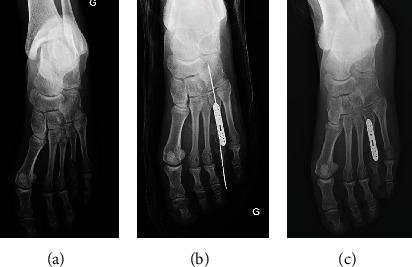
Case 2, a 26-year-old female with bilateral brachymetatarsia of the fourth toes who underwent surgical management of the left foot. (a) Preoperative X-ray of the left foot showing a shortness of the fourth toe of around 10 mm. (b) Postoperative X-ray of the left foot. (c) Post-operative X-ray after the K-wire removal at 1-month follow-up.
